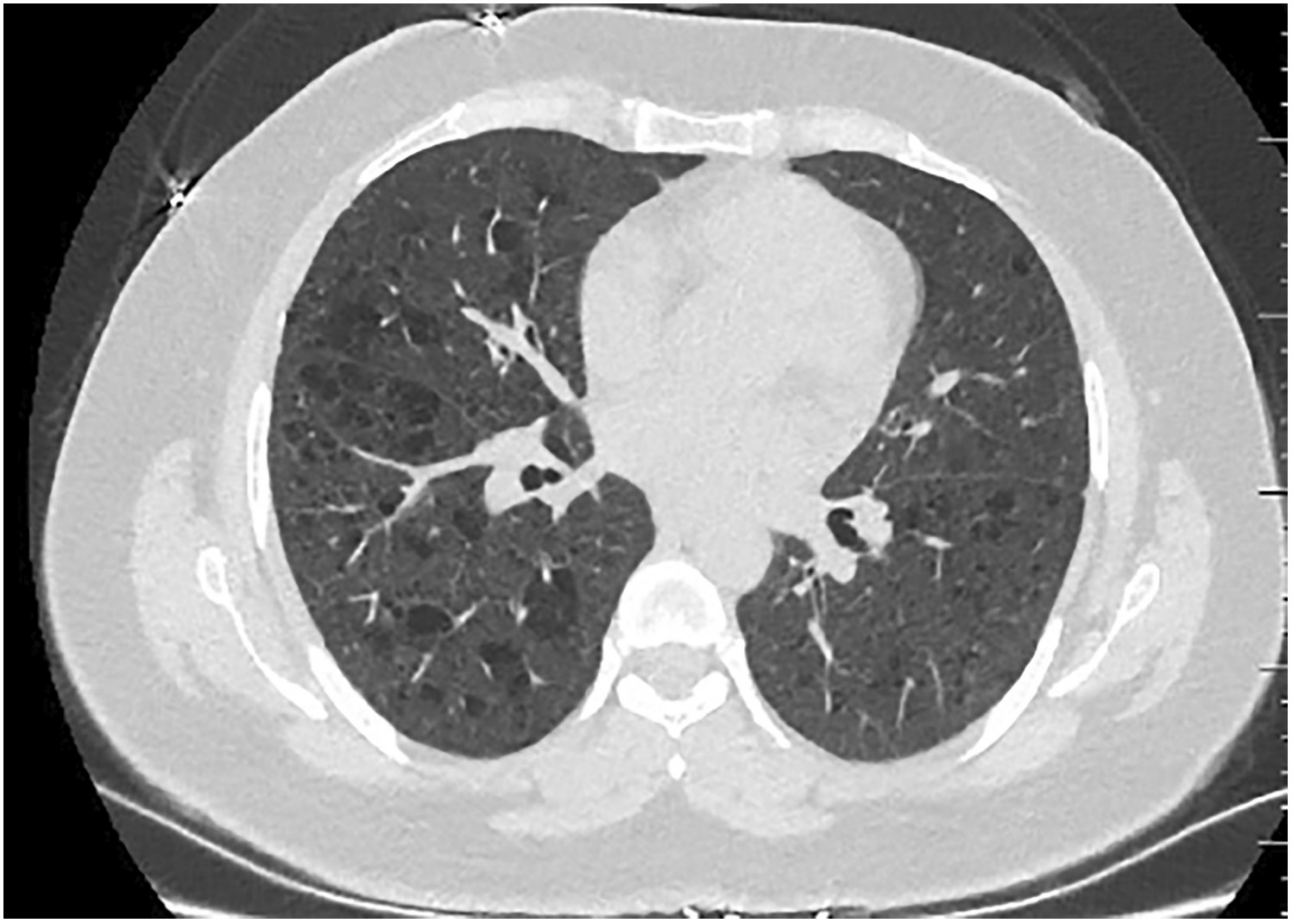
Cross‐sectional thoracic CT image of Case 1 (Image of thin‐walled air cysts and bleb).
